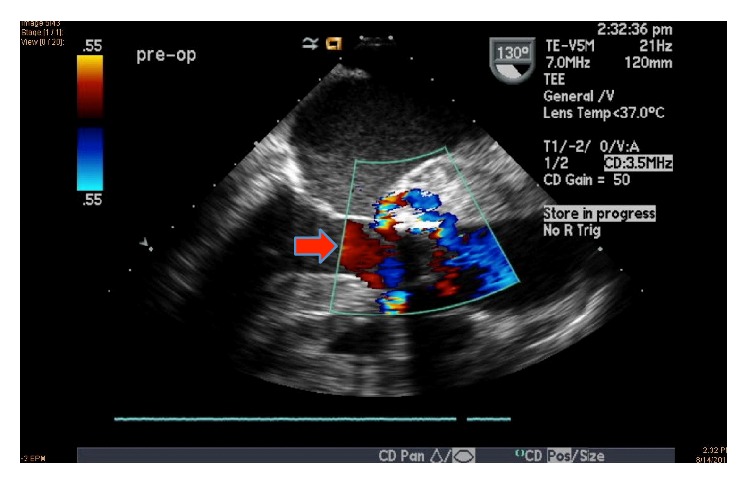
Transesophageal echocardiogram in long axis view with color flow Doppler showing severe paravalvular aortic regurgitation (indicated by red arrow), around a bioprosthetic aortic valve, around which an extensive aortic root abscess has developed.
